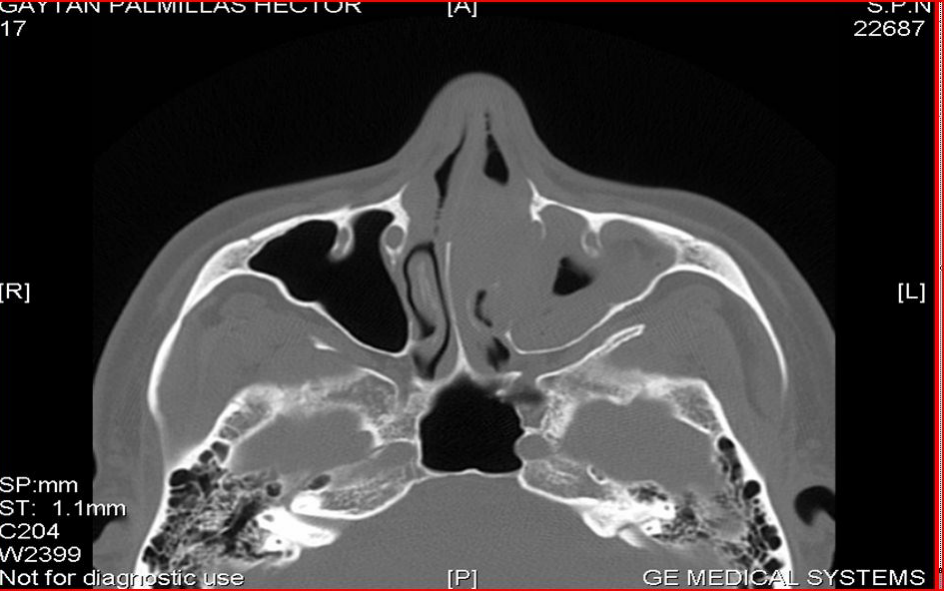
CT Scan, of paranasal sinus, that shows the tumor in the left nasal cavity.
